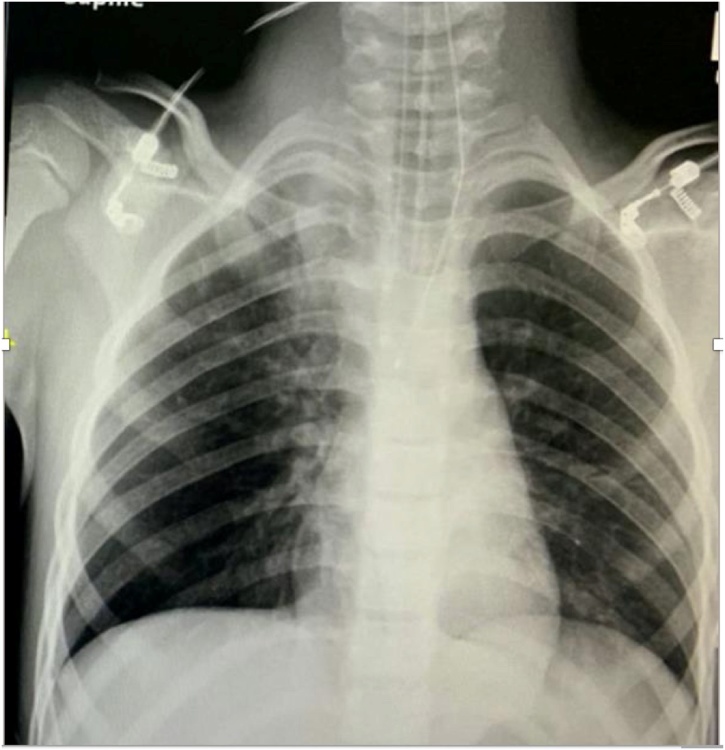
Repeated chest x-ray showing satisfactory ETT position and absence of pneumothorax.
